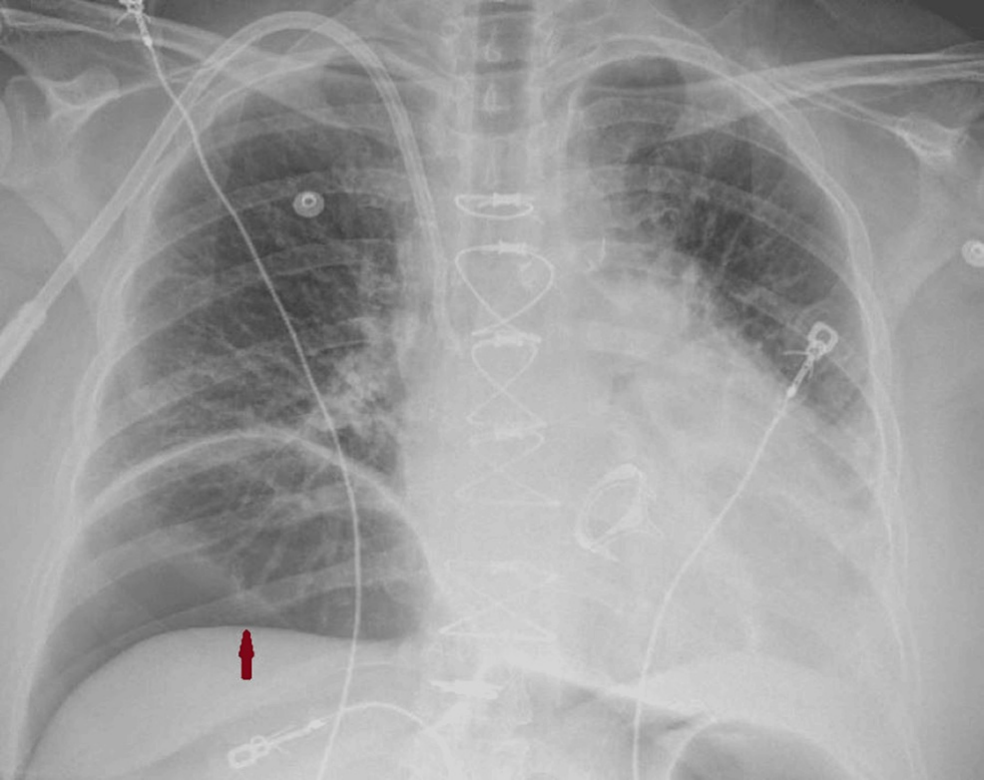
Supine chest X-ray demonstrating an area of lucency underneath the right hemidiaphragm representing intra-abdominal free air (red arrow)
